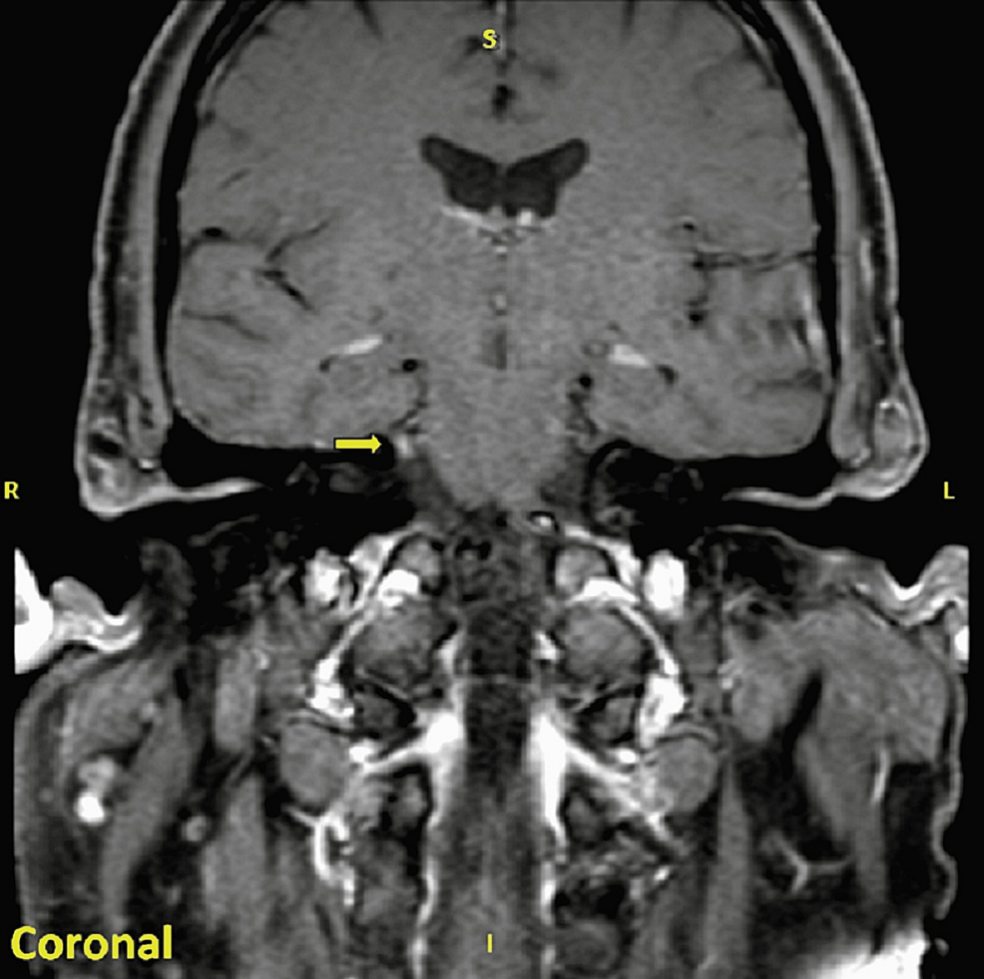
Brain MRI with IV Contrast, Trigeminal Nerve Enhancement T1 thin slice section of brain MRI with IV gadoterate meglumine showing nonspecific enhancement involving the cisternal segment of the right trigeminal nerve extending into Meckel's cave. MRI: magnetic resonance imaging.
